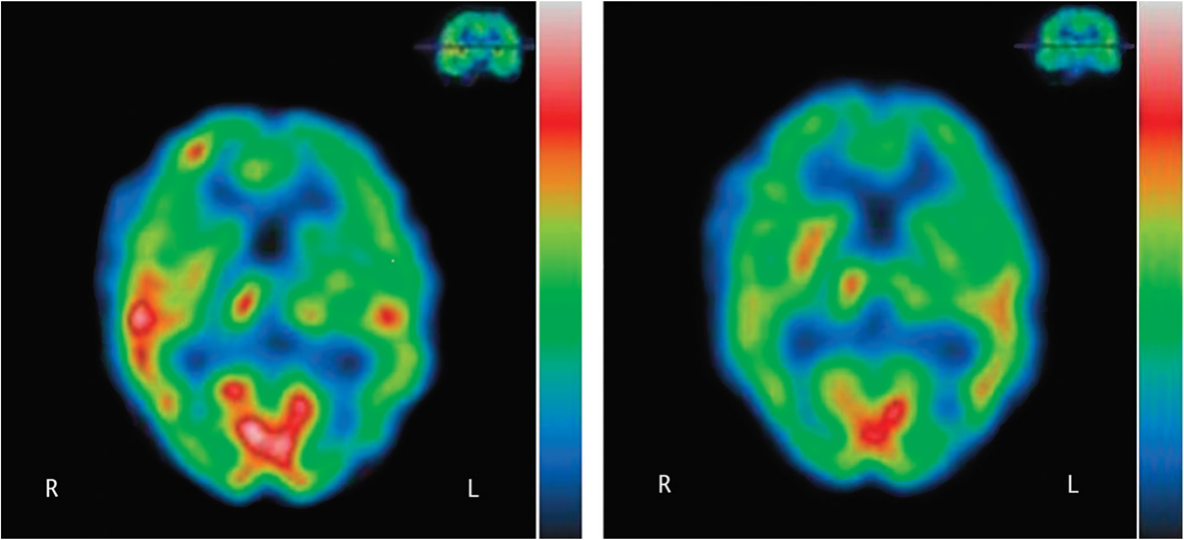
Single photon emission computed tomography (technetium-99 m-ethyl cysteinate dimer) images before (*left*) and after (*right*) treatment [24]. Hyperperfusion in the right relative to the left temporal lobe improved after treatment in parallel with the alleviation of the oral cenesthopathy
